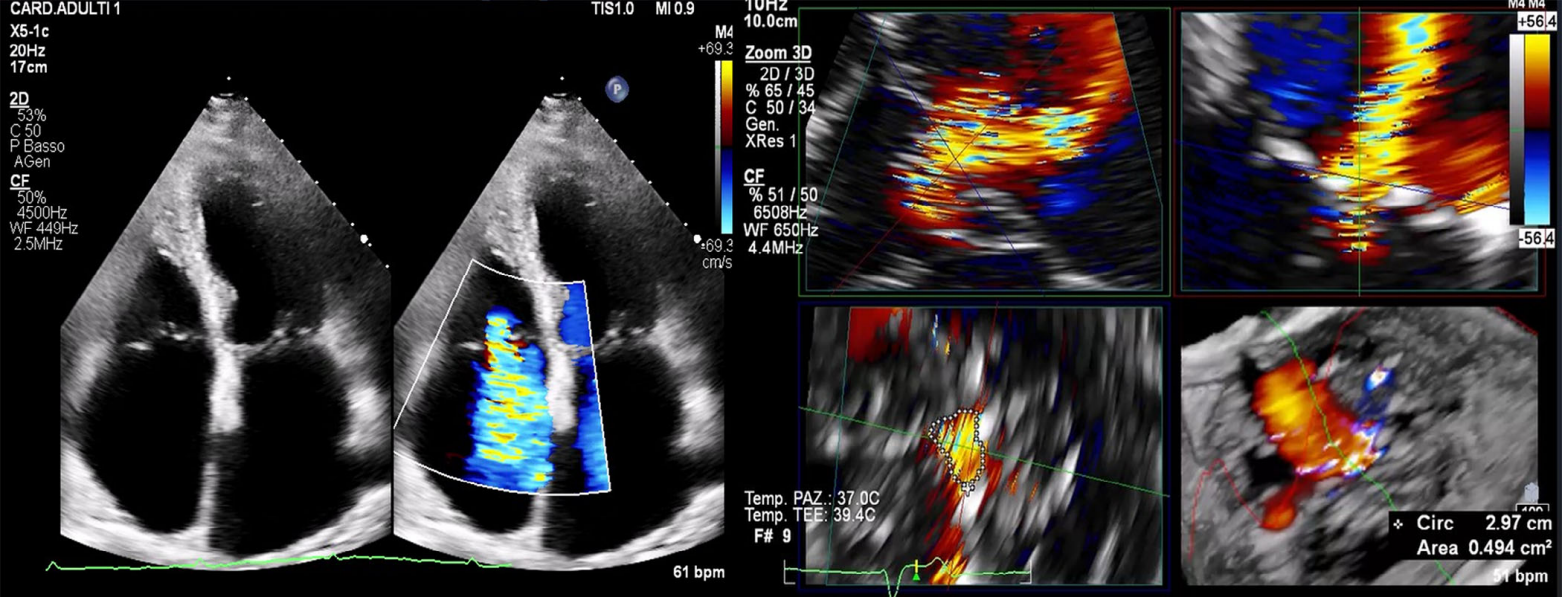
On the left: TTE four chambers view with and without color Doppler, optimal view to measure the 2 dimensional (2D) “VC”; On the right: TOE 3D reconstruction of 3D EROA. 2D indicates 2 dimensional; EROA, effective regurgitant orifice area; TOE, transesophageal echocardiography; TTE indicates transthoracic echocardiography; VC, vena contracta.
